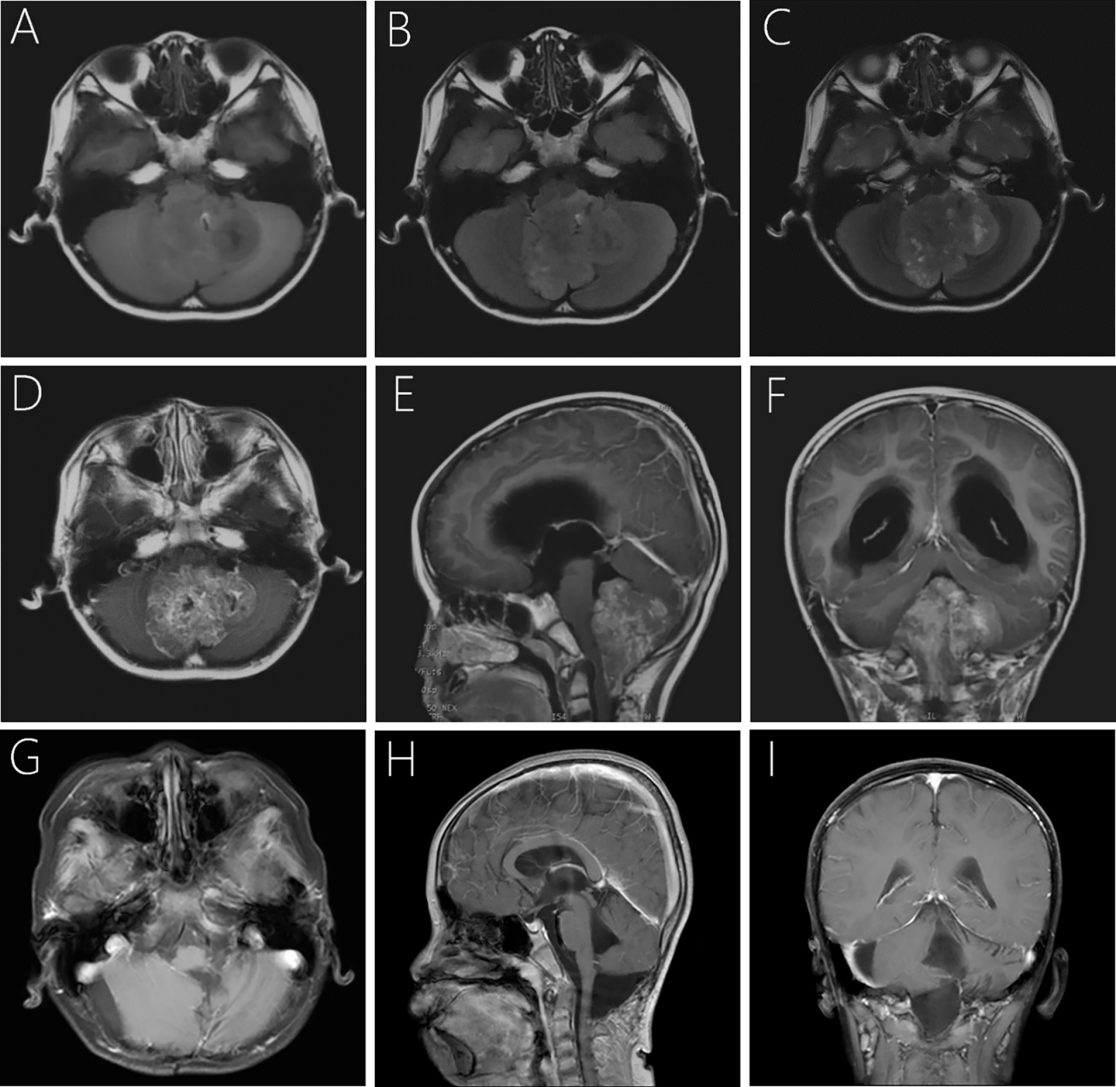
Pre- and postoperative imaging of the child. Preoperative MRI showed hypo- and isointensity mixed signal, together with focal hyperintensity on T1WI (A) and T2-weighted FlAIR (B). The lesion is iso- and hyperintensity mixing signals and well-circumscribed on axial T2WI (C). Post-contrast T1WI showed moderate heterogeneous contrast enhancement of the lesion (D–F). Follow-up MRI at 29-month after surgery revealed that the lesion was completely removed with no signs of recurrence (G–I).
